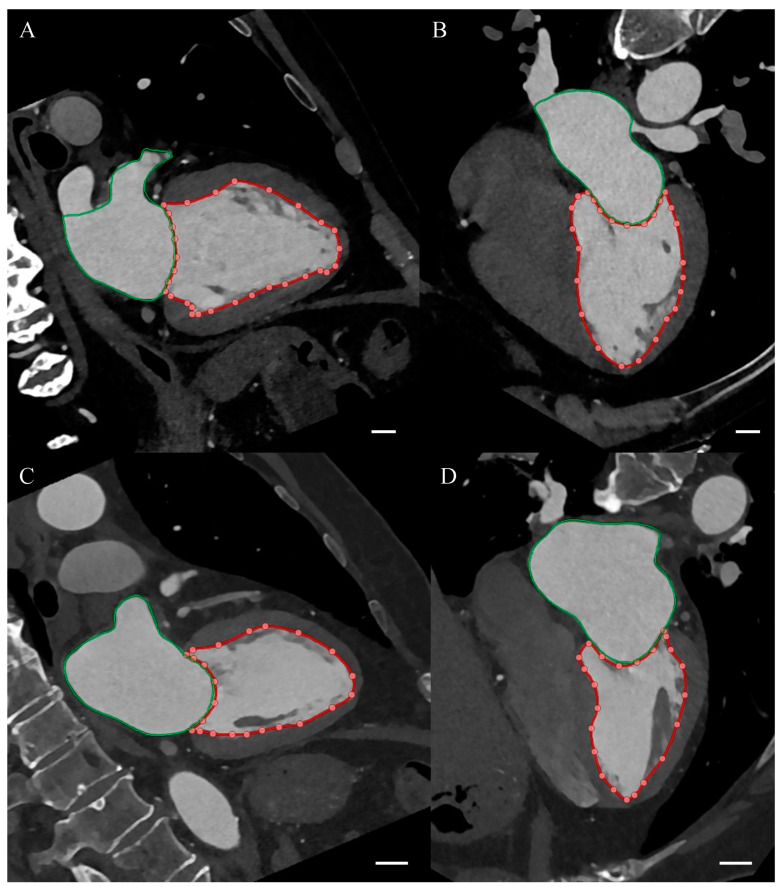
Cardiac computed tomography. The red curve with dots indicates the outline of the left ventricle, the green curve indicates the outline of the left atrium. The white section in the lower right corner of each panel is 1 cm long on the image magnification scale. (A) Two-chamber view in a patient with a left atrioventricular coupling index (LACI) of 21%. (B) Four-chamber view in a patient with LACI of 21%. (C) Two-chamber view in a patient with LACI of 56%. (D) Four-chamber view in a patient with LACI of 56%.
